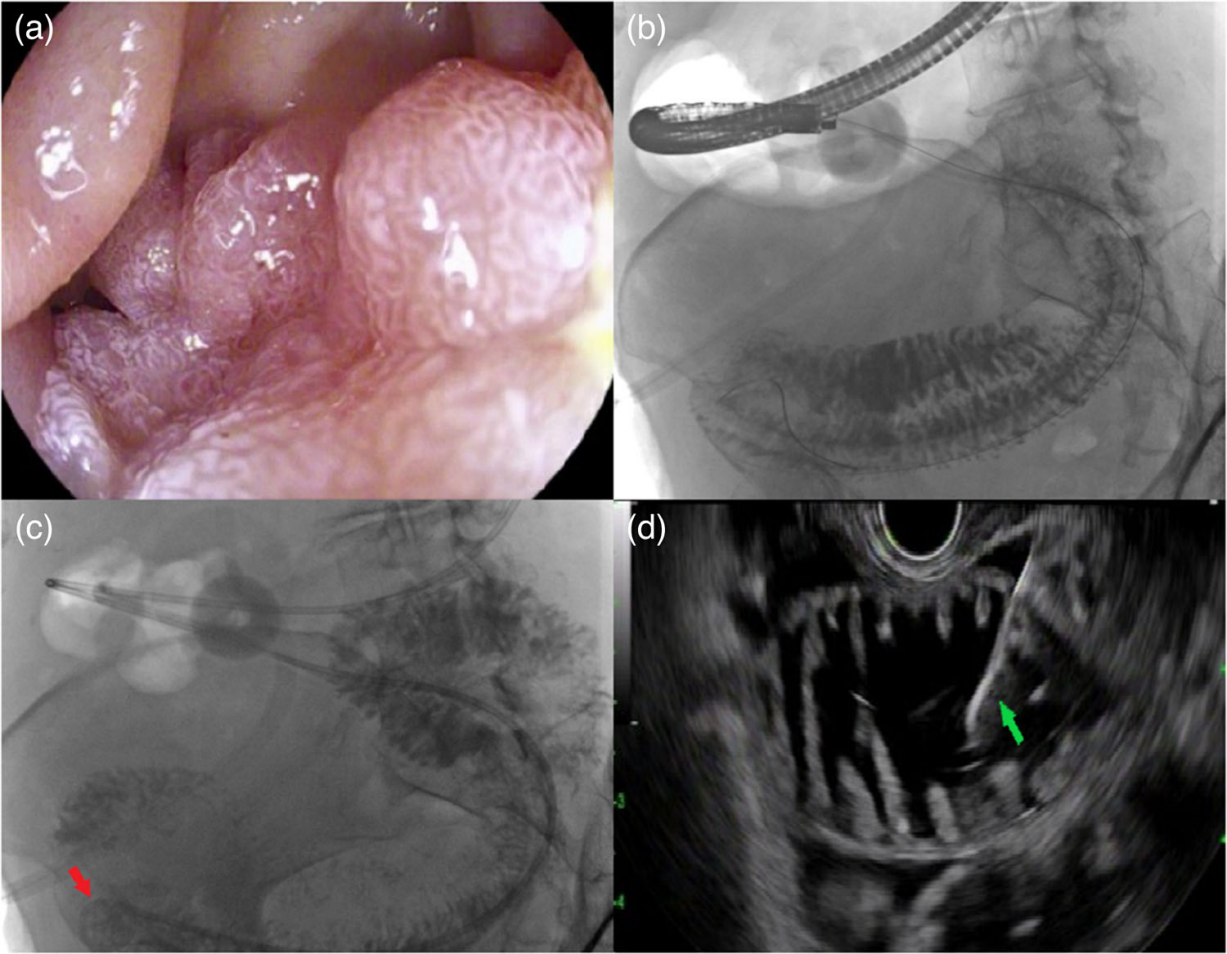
(a) Diagnostic upper endoscopy demonstrating duodenal adenocarcinoma with resultant luminal narrowing. (b) Fluoroscopic image of a Visiglide 2 wire traversing a duodenal stricture with contrast opacifying the small bowel. (c) Nasocystic catheter placement with the tip (red arrow) distal to the ligament of Treitz in the jejunum. (d) Endoscopic ultrasound image showing a distended loop of small bowel during successful fine needle aspiration of blue‐tinged fluid with a 22‐G fine needle aspiration needle.
